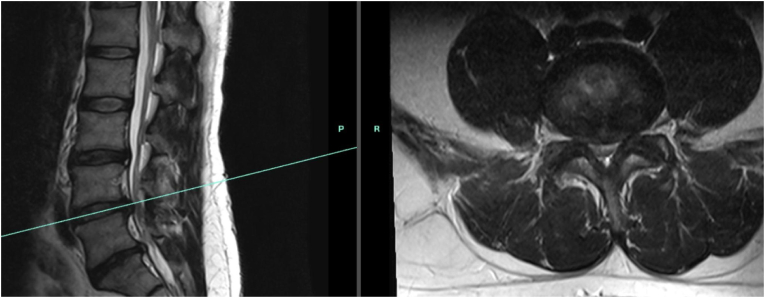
Outpatient MRI lumbar spine without contrast prior to hospital presentation.
